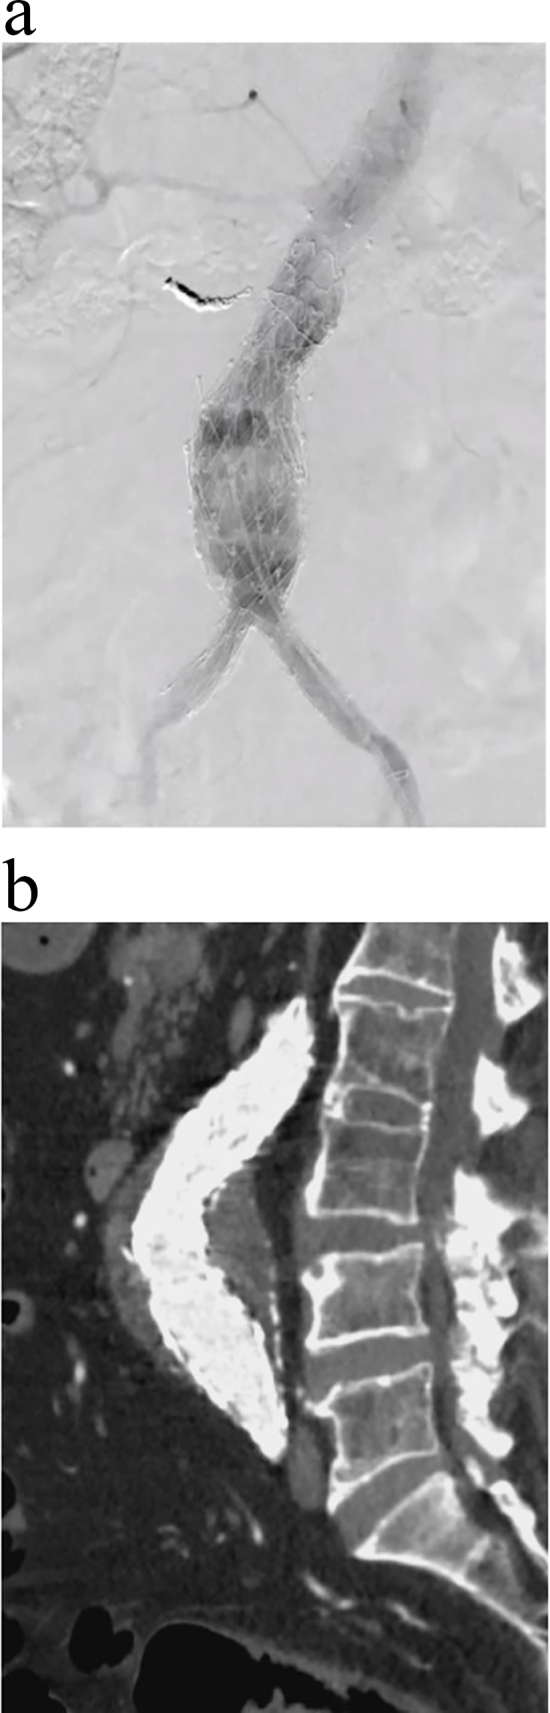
(A) No endoleaks are observed on completion of angiography. (B) Postoperative computed tomography (CT) scan.
